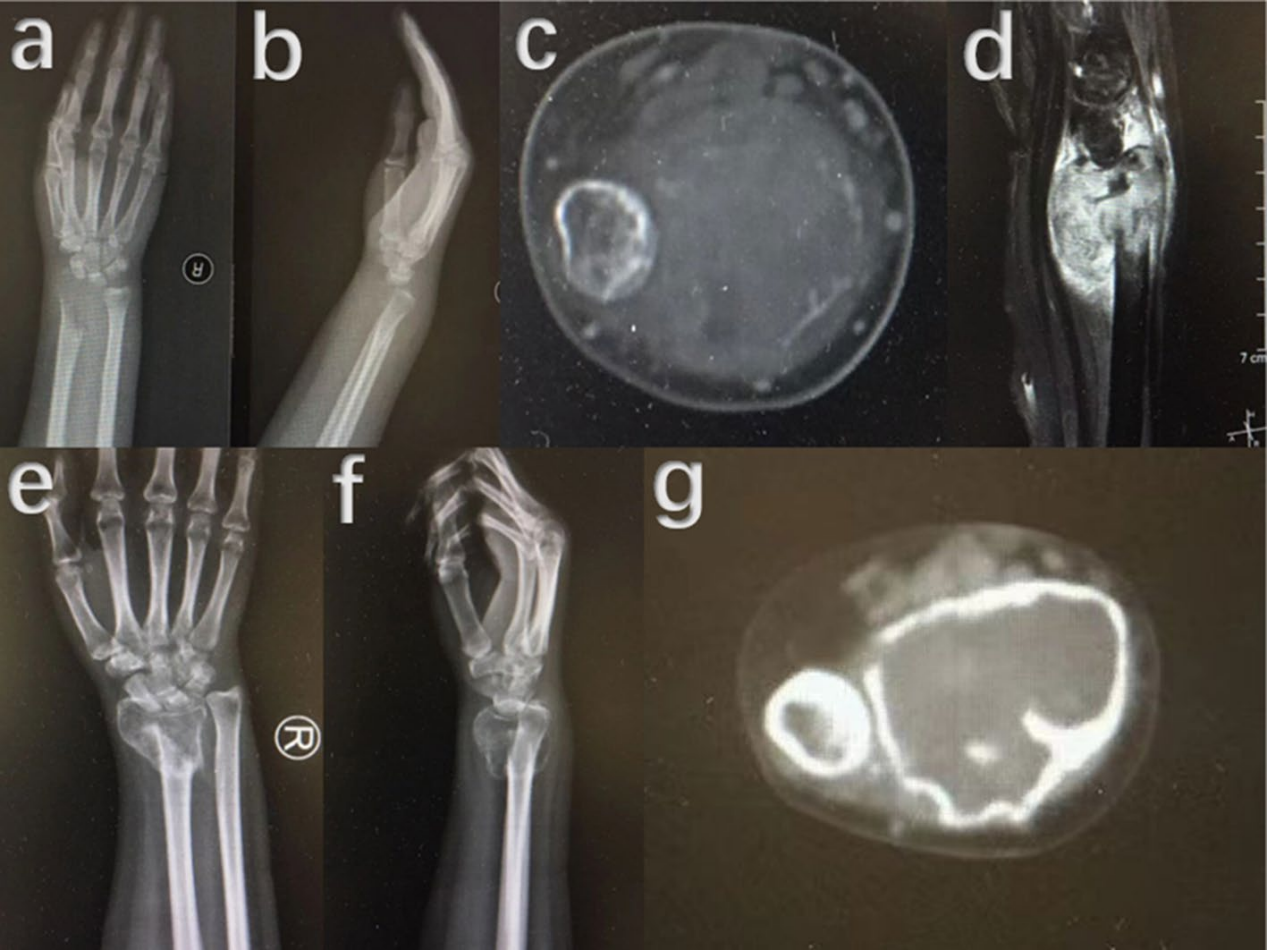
Preoperative images of another 22 years old female patient with GCTB located in distal radius. a–d Osteolytic destruction in right distal radius were revealed by X-ray (a, b), CT (c) and MRI (d). e–g Ossification could be observed on X-ray radiography (e, f) and CT (g) after application of denosumab for 2 months
